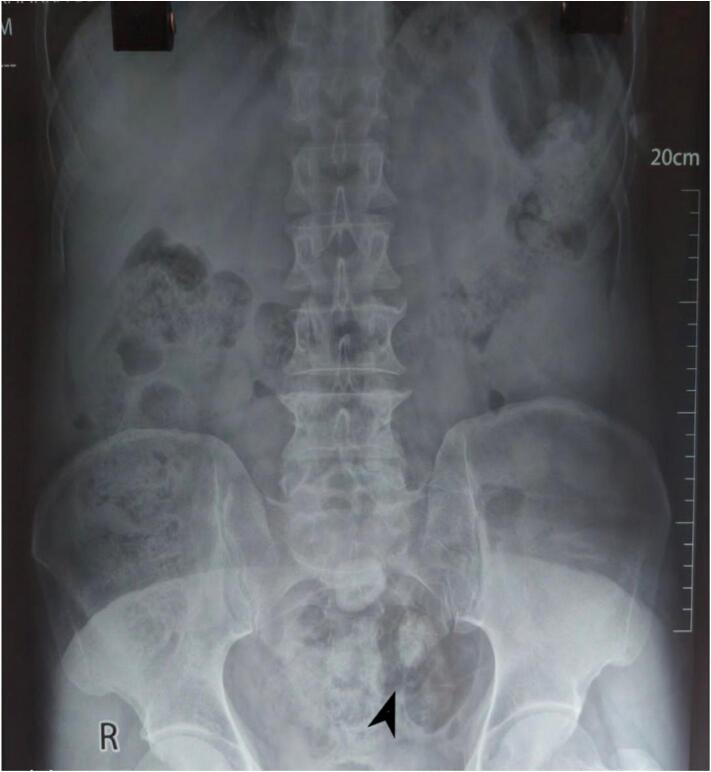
Erect plain abdominal radiograph showing a round radiopaque shadow (black arrow) suggestive of enterolith.
